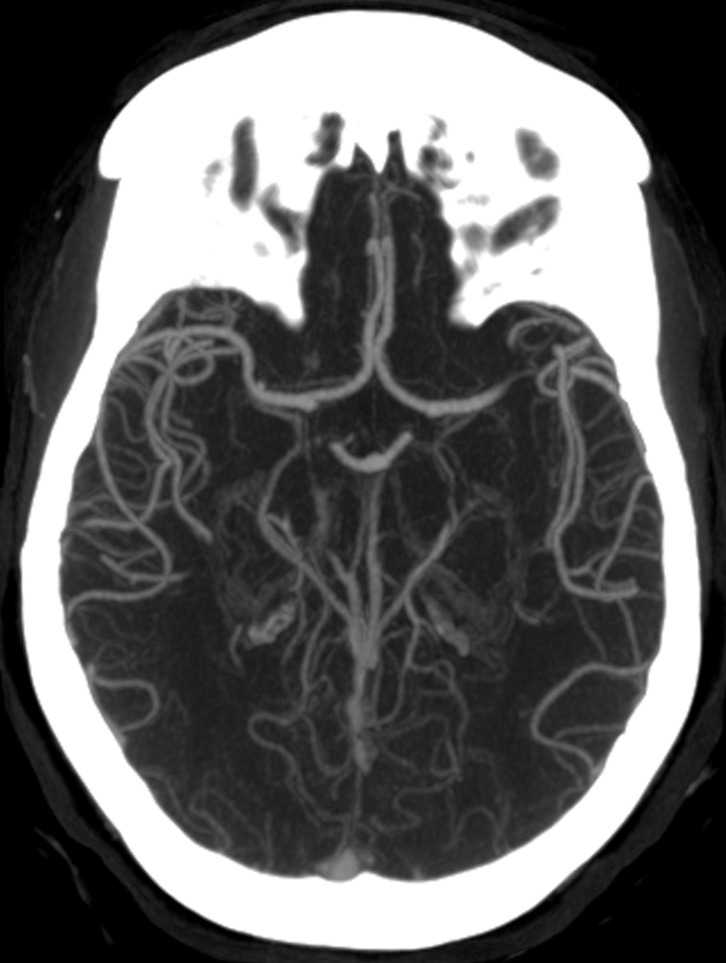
Axial computed tomography angiogram of the head performed with excellent technique through the region of the anterior communicating artery. Despite rigorous scrutiny by a neuroradiologist, no aneurysm was present.
